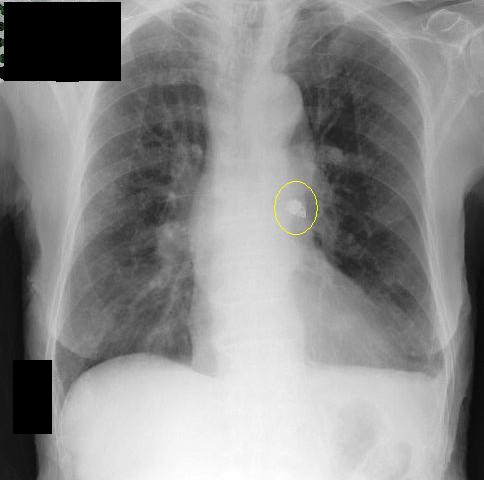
X-ray of the chest. X-ray of the chest confirming the presence of the capsule in the left side of the bronchopulmonary tree.
